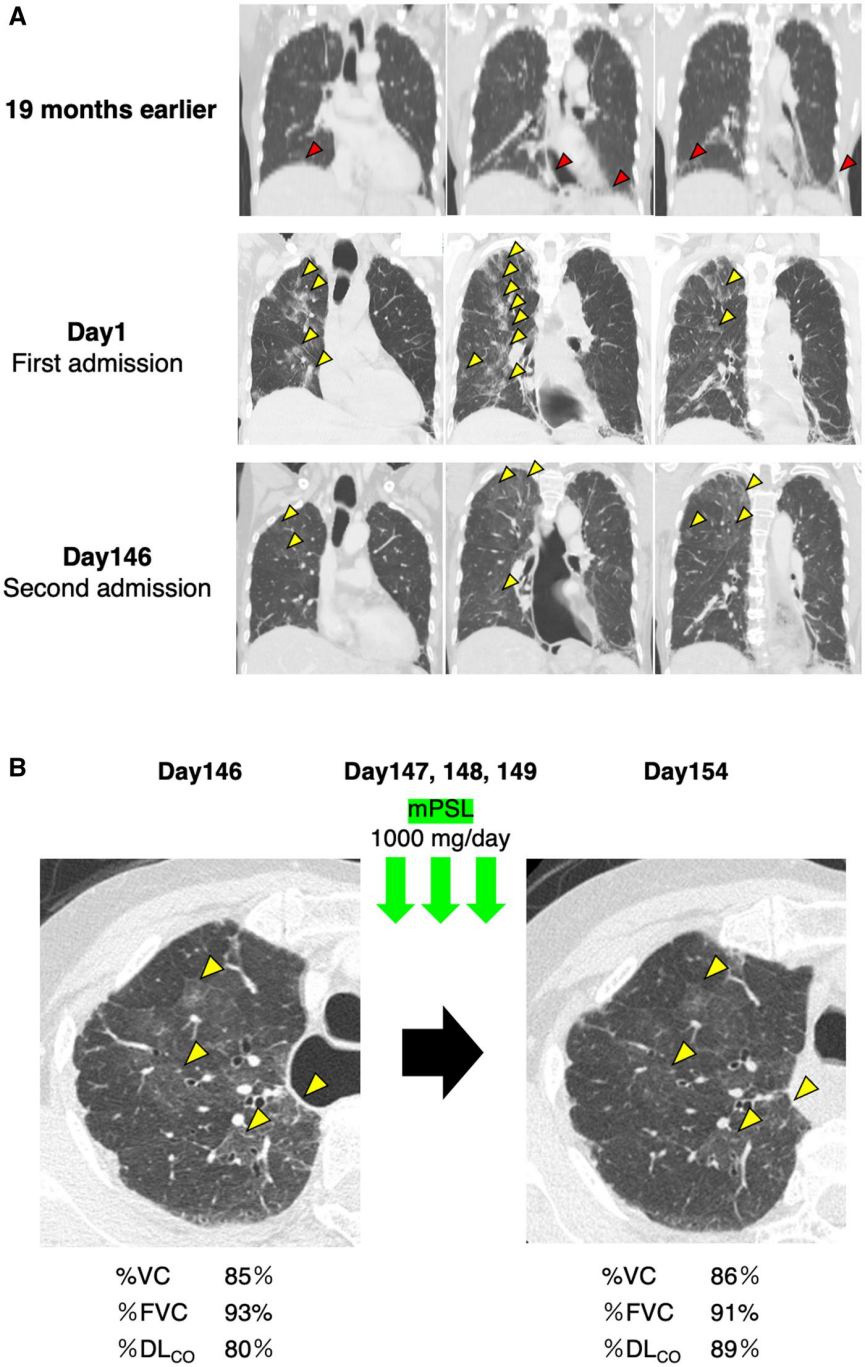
Clinical course of the presented case. (A) Coronal high-resolution computed tomography (HRCT) images at 19 months earlier, and on first and second admission. The time of the first admission was defined as day 1. There were mild subpleural reticular shadows at the bilateral lung bases, consistent with nonspecific interstitial pneumonia, on the baseline (arrowheads in the first raw). On admission, there were numerous panlobular ground-glass opacities with intralobular reticular opacities (crazy-paving appearance) throughout the lungs (arrowheads in the second and the third raws). (B) Comparison of horizontal HRCT images and lung function before and after mPSL pulse therapy during the second admission. Panlobular ground-glass opacities with intralobular reticular opacities improved following mPSL pulse therapy (arrowheads)
